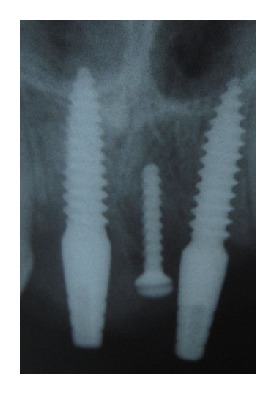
postoperative radiograph showing implant and screw.
